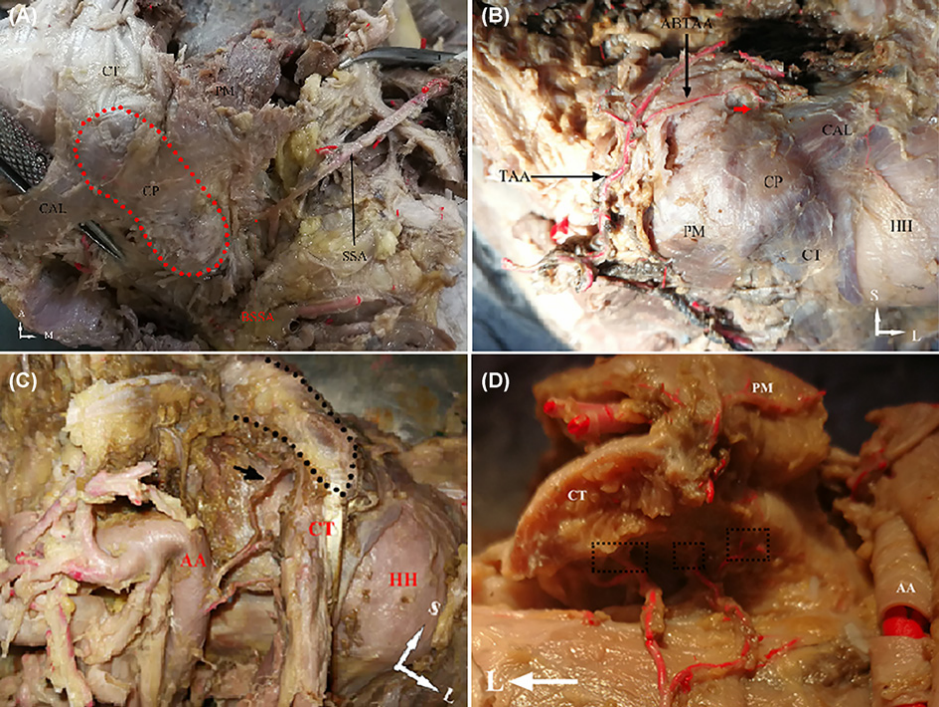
Macro observation of arterial supply of CP (A) Superior aspect of CP and SSA (left shoulder). (B) Superior aspect of antero-superior part of CP and its adjacent structures (left shoulder), the red thick arrow shows the branches of ABTAA. (C) Internal aspect of CP and CT with PM removed (left shoulder), the black thick arrow points to the branch that supplies the inferior side and the black dot outlines the CP. (D) Inferior aspect of CP with CT removed (right shoulder). The black dotted squares show the vessels that supply the inferior side. Abbreviations: BSSA, branch of SSA; HH, humeral head.
